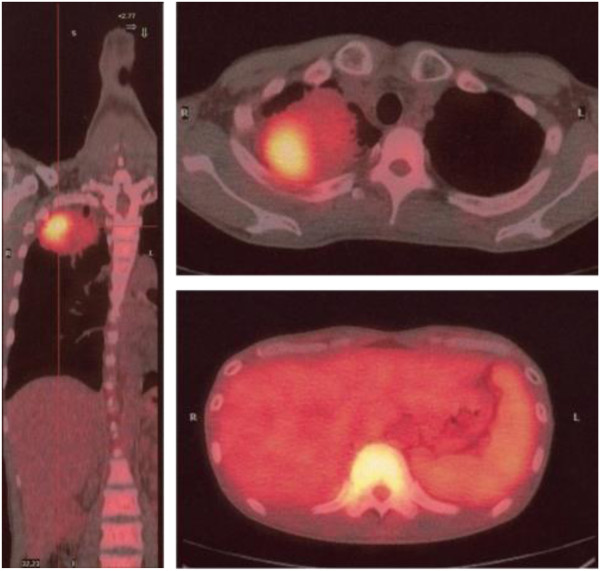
FDG-PET/CT in September 2005. FDG-PET/CT showed the localized uptake of FDG to the center of the mass-like lesion, in addition to diffuse uptake by the bone marrow and an enlarged liver and spleen.
